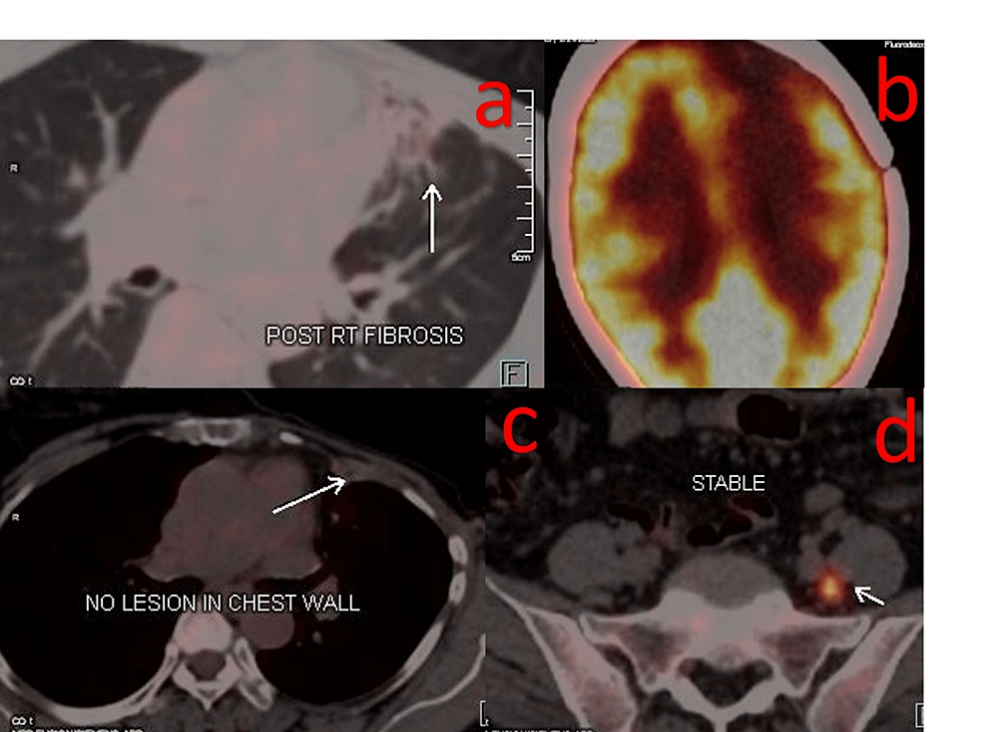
PET-CT scan report post 28 cycles of T-DM1 in February 2023 suggestive of stable disease and no lesion in the chest wall (c, d). Post-RT fibrosis and post-RT changes (a, b).
